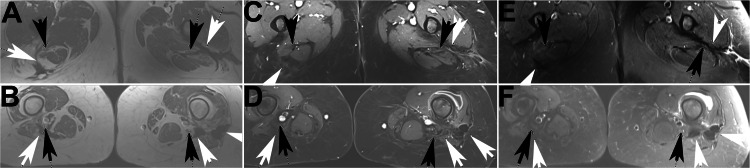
Sequential axial MR images of Case 1, continuation from Figure 2 Left column (A, B): T1 sequence; middle column (C, D): T1 fat saturation sequence post contrast; right column (E, F): T2 fat saturation sequence. Black arrows indicate sciatic nerve; white arrows indicate tumor
